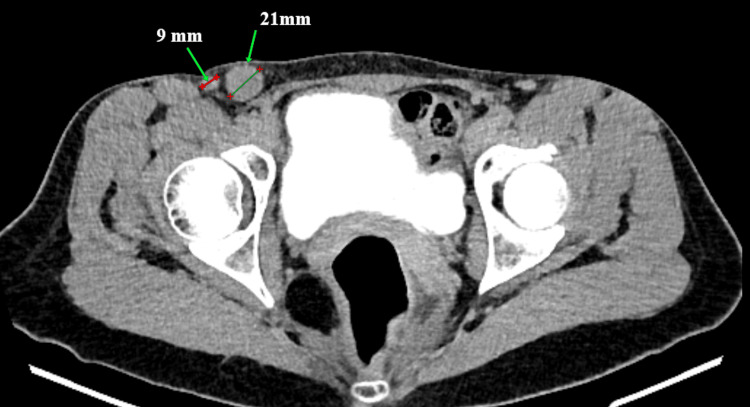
Pre-treatment computed tomography scan demonstrating the enlarged right inguinal lymph nodes Axial computed tomography image demonstrating multiple enlarged and structurally altered right inguinal lymph nodes prior to neoadjuvant chemotherapy. The largest lymph node measured up to 21 mm, consistent with metastatic involvement.
